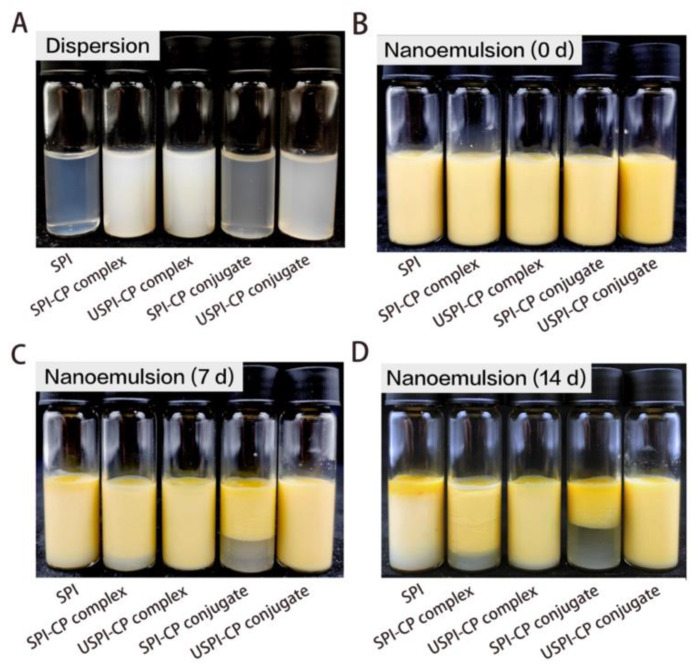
Images of original dispersions of SPI, SPI–CP complex, USPI–CP complex, SPI–CP conjugate and USPI–CP conjugate, respectively (A), and images of β-carotene nanoemulsions stabilized by different emulsifiers stored at 25 °C without direct light irradiation for 0 d (B), 7 d (C) and 14 d (D), respectively. Samples: SPI, soy protein isolate; SPI–CP complex, electrostatic complex formed between soy protein isolate and citrus pectin; USPI–CP complex, electrostatic complex formed between soy protein isolate and citrus pectin with ultrasound treatment; SPI–CP conjugate, Maillard-type conjugate formed between soy protein isolate and citrus pectin; USPI–CP conjugate, Maillard-type conjugate formed between soy protein isolate and citrus pectin with ultrasound treatment.
